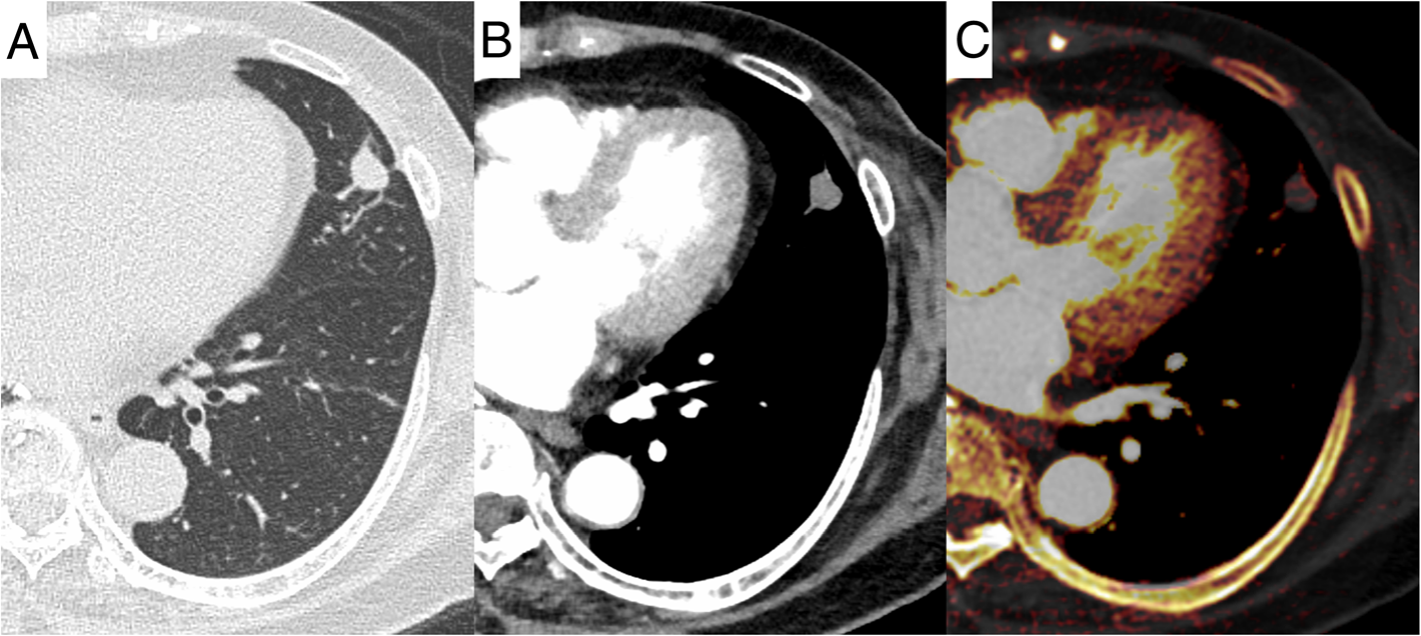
A 74-year-old female patient. A poorly-differentiated (G3) adenocarcinoma is observed in the left lingula. Vascular invasion, and pleural involvement were positive. a Lung window setting. b Mediastinal window setting. c Iodine-enhanced image of contrast-enhanced CT. The 3D-IRA is 16 HU, and the corrected 3D-IRA is 2.85
